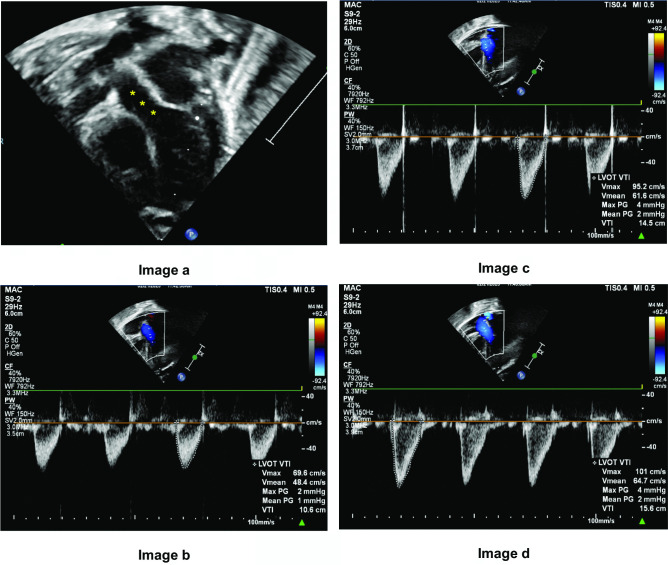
Measurement of VTI at different locations along the LVOT. a shows VTI measured at the 3 locations along LVOT in apical 5 chambers view (yellow asterisks). b–d show changes in VTI measurement as location of Doppler sampling gate changes. b shows VTI sampled below the valve. c shows VTI sampled at the hinges of valve. d shows VTI sampled at the tip of the valve. VTI, velocity time integral. LVOT, left ventricular outflow tract
